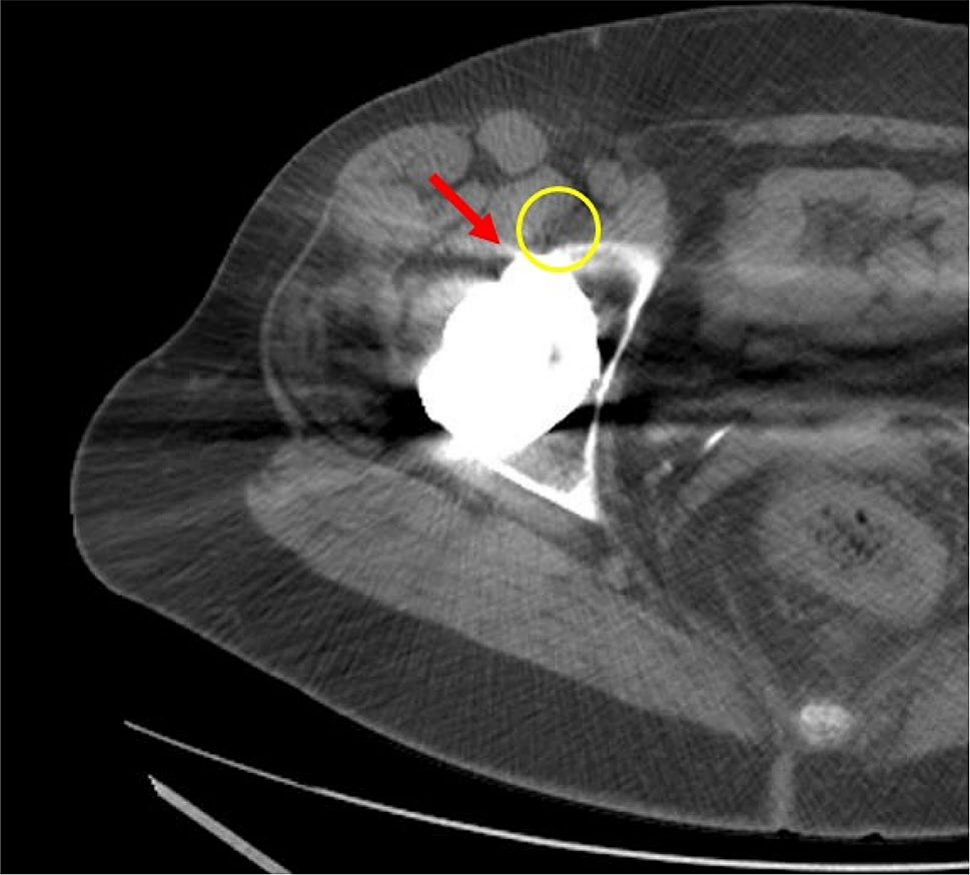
Axial CT scan of a right hip, red arrow: prominent acetabular cup; yellow circle: iliopsoas tendon irritated by acetabular cup prominence
